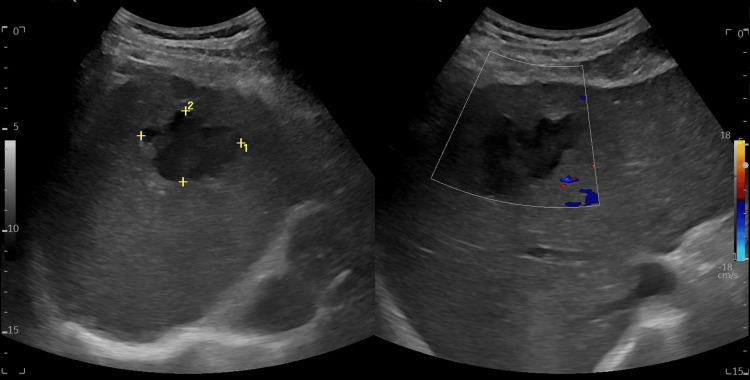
Ultrasound images showing a heterogeneous hypoechoic lesion in the right hepatic lobe with internal echoes and absence of posterior acoustic enhancement, without internal vascularity on Doppler, compatible with hepatic abscesses.
